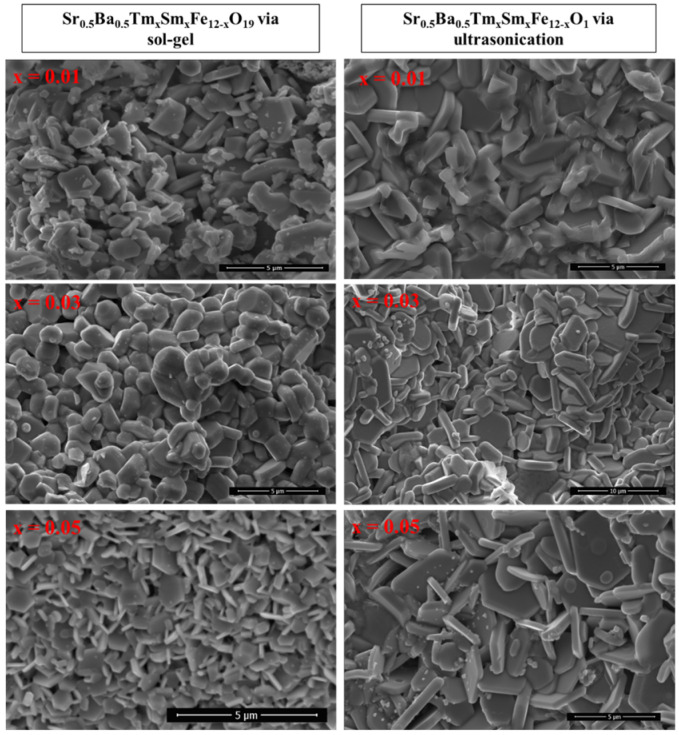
SEM images of Sr_0.5_Ba_0.5_Tm_x_Sm_x_Fe_12−2x_O_19_ (x = 0.01, 0.03, and 0.05) hexaferrites prepared using sol–gel auto-combustion (left) and ultrasonication (right) techniques.
